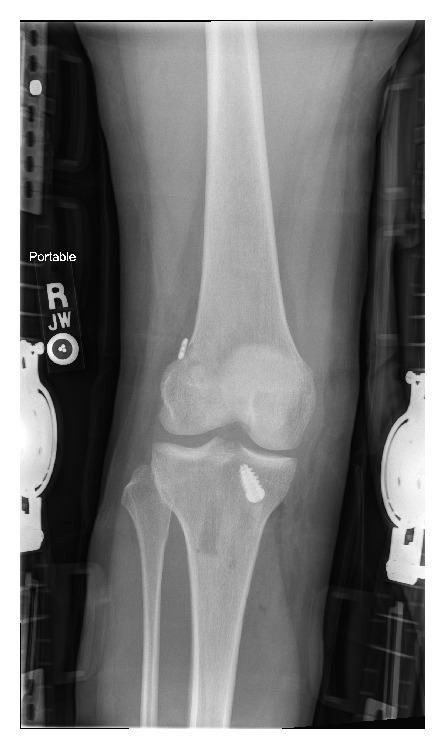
Immediate ACL reconstruction postoperative radiographs in AP view.
